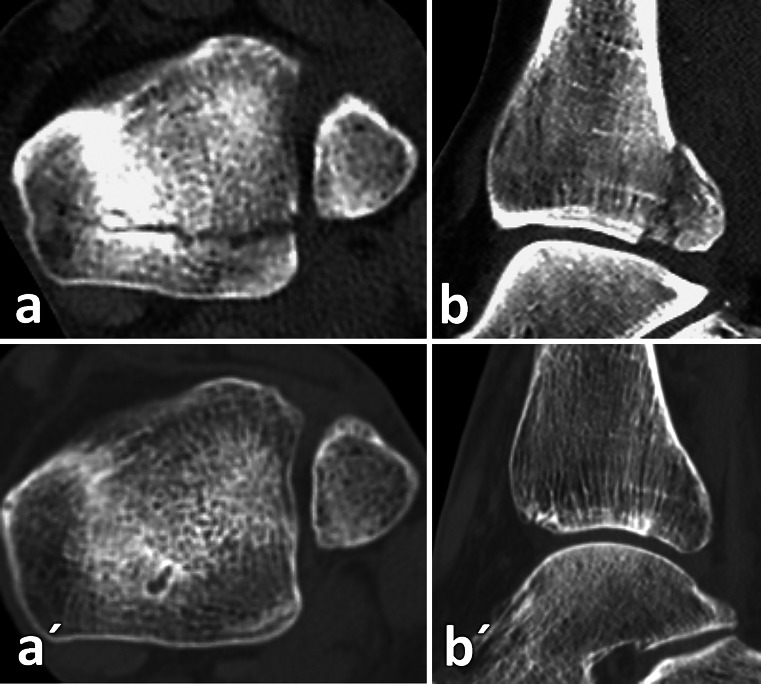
Patient N-13. a – an axial postinjury CT scan fracture shows 10 degrees of external rotation of the distal fibula in FN and fracture of PM type 3 according B-R classification; b – a sagittal postinjury CT scan with 2 mm proximal displacement of PM; a´ - an axial follow-up CT scan 12 months after injury, external rotation of the distal fibula in FN is smaller; b´- a sagittal follow-up CT scan 12 months after injury fracture shows healing of PM in an almost anatomical position
